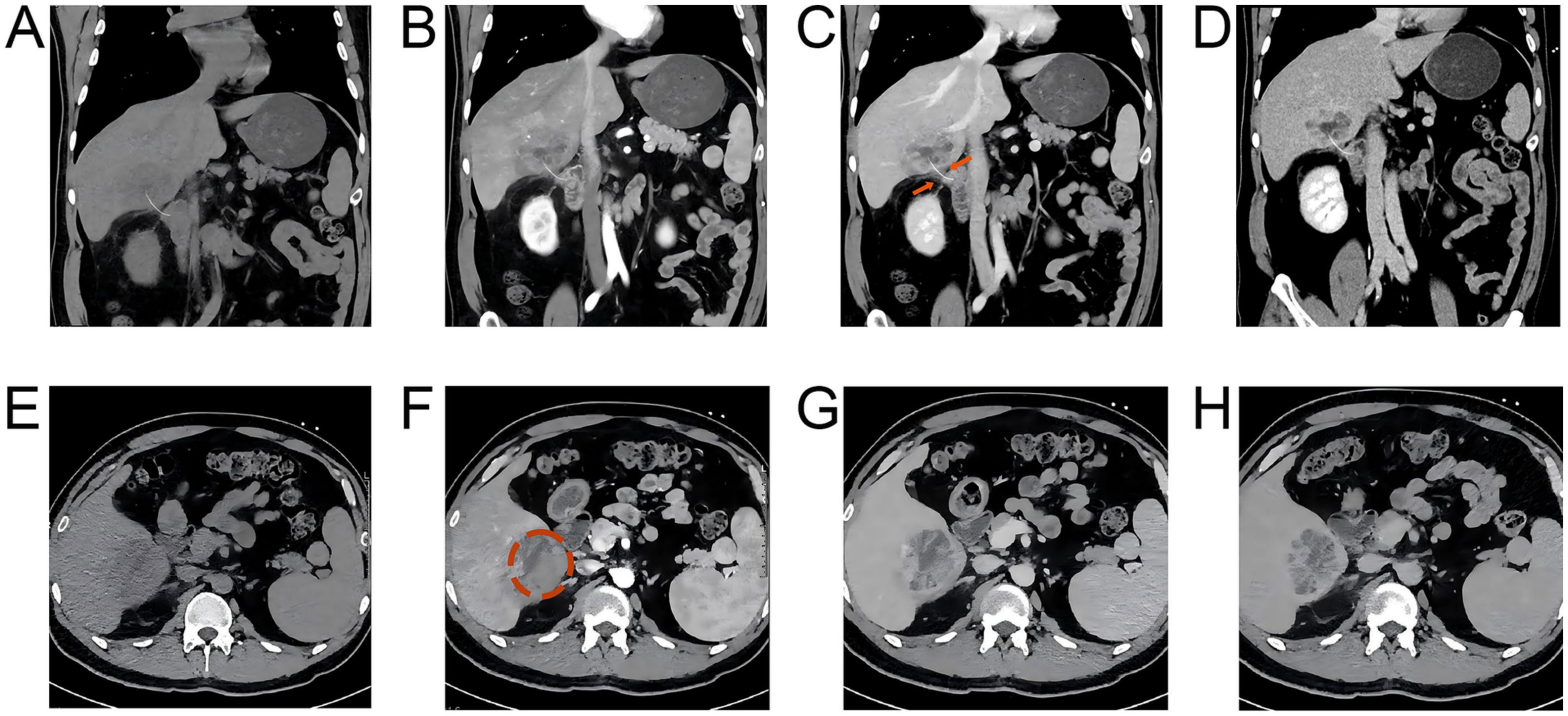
Computed tomography (CT) scan images. (A) Non-contrast coronal view: an arc-shaped linear hyperdense lesion (foreign body, fish bone) was identified with its two ends located in the right hepatic lobe and the descending duodenum, respectively. A corresponding massive hypodense lesion (hepatic abscess) was observed in the right hepatic lobe. Mild exudative changes were noted in the space between the descending duodenum and the right hepatic lobe. (B) Arterial phase coronal view: the hepatic abscess in the right hepatic lobe showed heterogeneous marked enhancement. An irregular hypodense area was visualized within the abscess, predominantly around the foreign body. Extensive hyperenhancement was detected in the hepatic parenchyma surrounding the abscess. (C) Portal venous phase coronal view: the hepatic abscess in the right hepatic lobe exhibited enhancement of the thick-walled septa. No significant enhancement was observed in the irregular hypodense area inside the abscess. The upper orange arrow indicates the hyperdense foreign body, and the lower orange arrow indicates the incompletely formed sinus tract surrounding the foreign body and associated periduodenal inflammatory changes. (D) Hepatic parenchymal phase coronal view: the thick-walled septa of the hepatic abscess in the right hepatic lobe showed density similar to the surrounding hepatic parenchyma, while no obvious enhancement was noted in the abscess cavity. (E–H) Non-contrast axial views, non-contrast and dynamic contrast-enhanced images: a hypodense lesion was found in the right hepatic lobe on the non-contrast scan. The lesion presented progressive enhancement of the thick wall and multilocular septa on contrast-enhanced scans, with no significant enhancement in the internal abscess cavity. The abscess cavity is indicated by the orange circle. (F) Extensive hyperenhancement was shown in the hepatic parenchyma surrounding the lesion.
